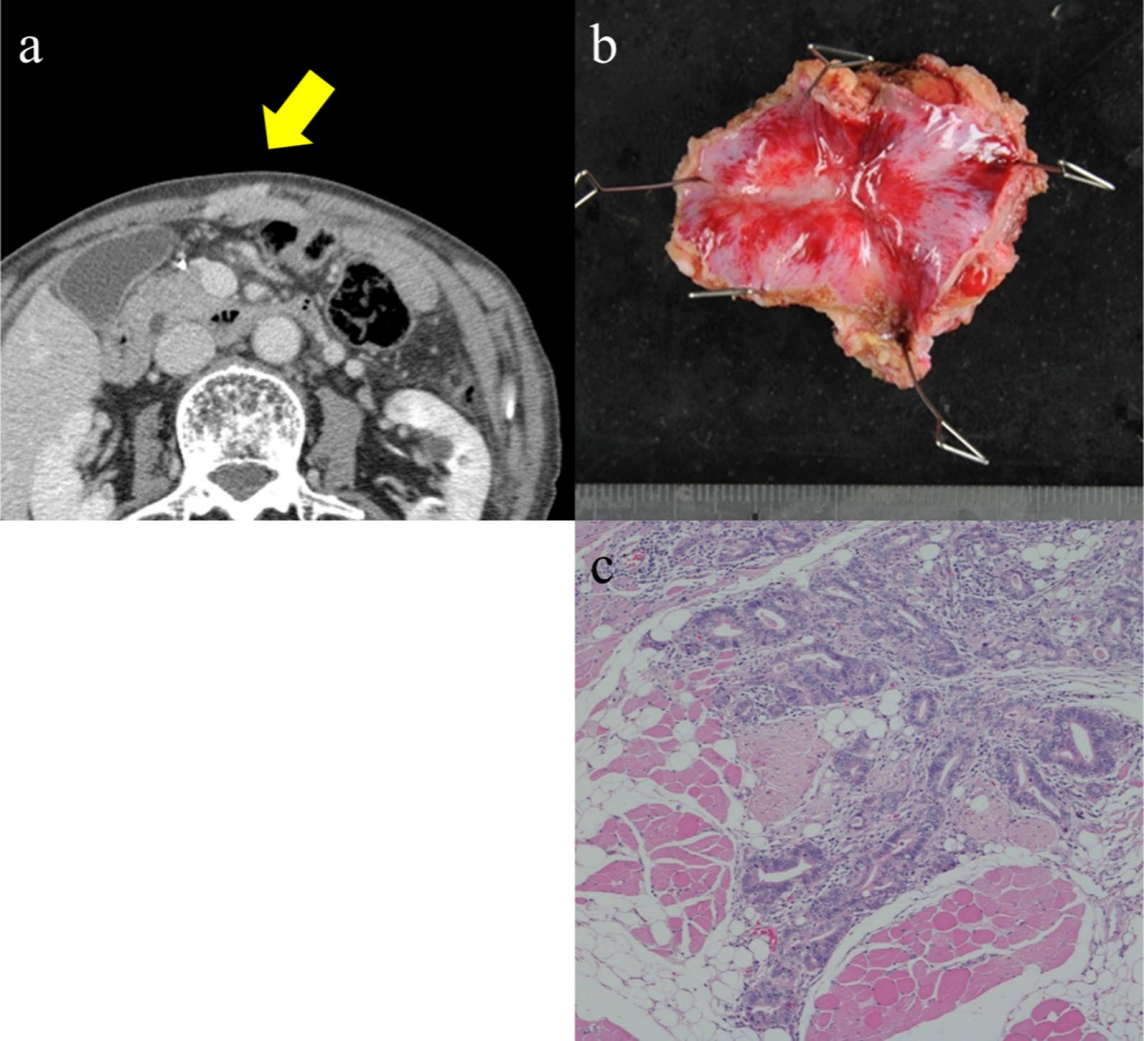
Computed tomography (CT) scan and pathological findings of the first recurrence. a CT scan shows an enhanced mass in the median line of abdominal wall. b Macroscopic findings show a white nodular lesion in the peritoneum. c Pathological examination reveals an adenocarcinoma consistent with metastatic tumor from gastric cancer
